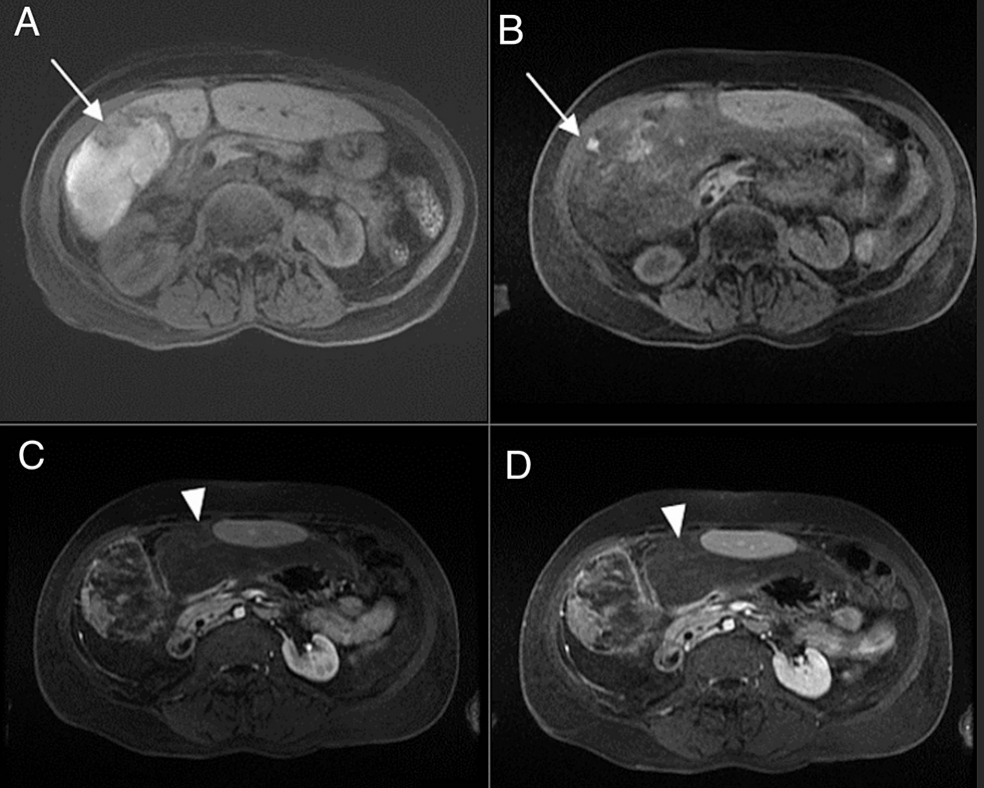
Representative images from follow-up MRI. A) Axial pre-contrast T1-weighted MRI showing nodularity attributed to possible ischemic changes from recent right hepatic artery embolization (white arrow) with subjacent intrinsic bright signal in the subhepatic mass consistent with hematoma. Post-contrast T1-weighted MRI images with arterial (B), portal venous (C), and delayed (D) phases showing abnormal round focus of enhancement and questionable enhancement within the adjacent hematoma suggestive of possible malignancy versus post-embolization changes near the right lobe (white arrow) surrounded by extensive non-enhancing material underneath the left lobe (white arrowhead), also consistent with hematoma. MRI: magnetic resonance imaging.
